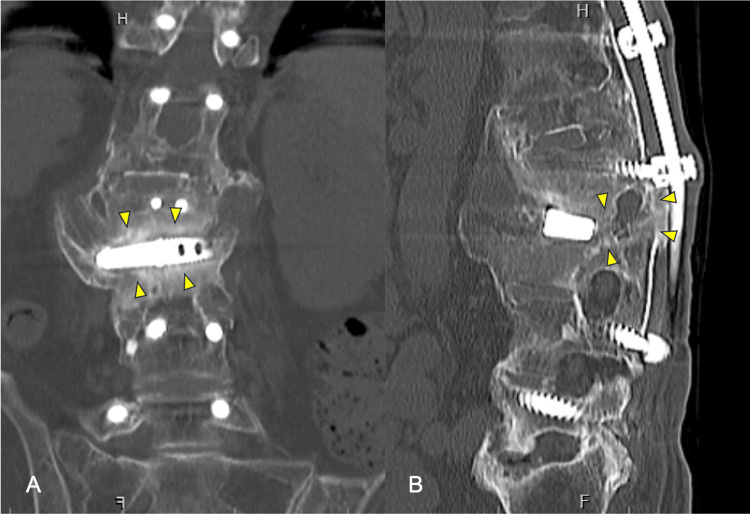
CT coronal (A) and sagittal (B) images showing bony fusion between L2 and L3 vertebral bodies at the six-month postoperative visit (yellow arrows).
